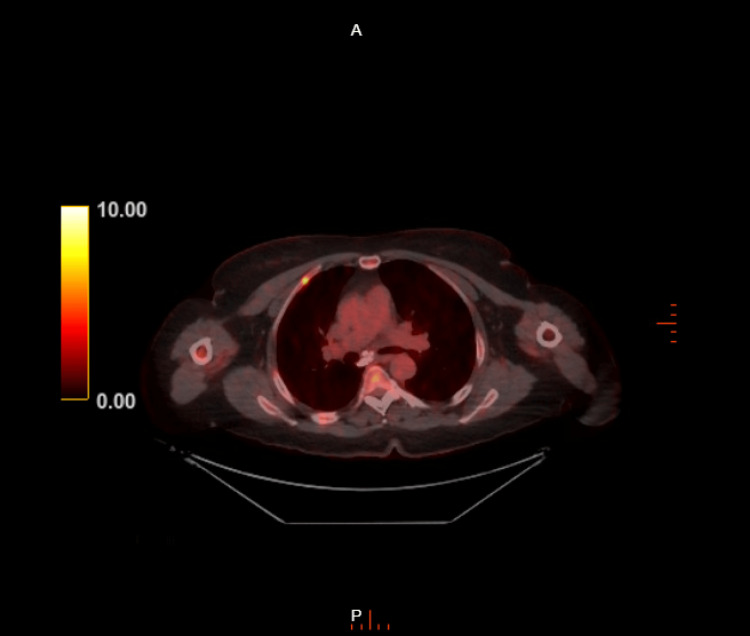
Positron emission tomography CT scan with FDG-avid lesion in the T5 vertebral body FDG: fluorodeoxyglucose, CT: computed tomography
